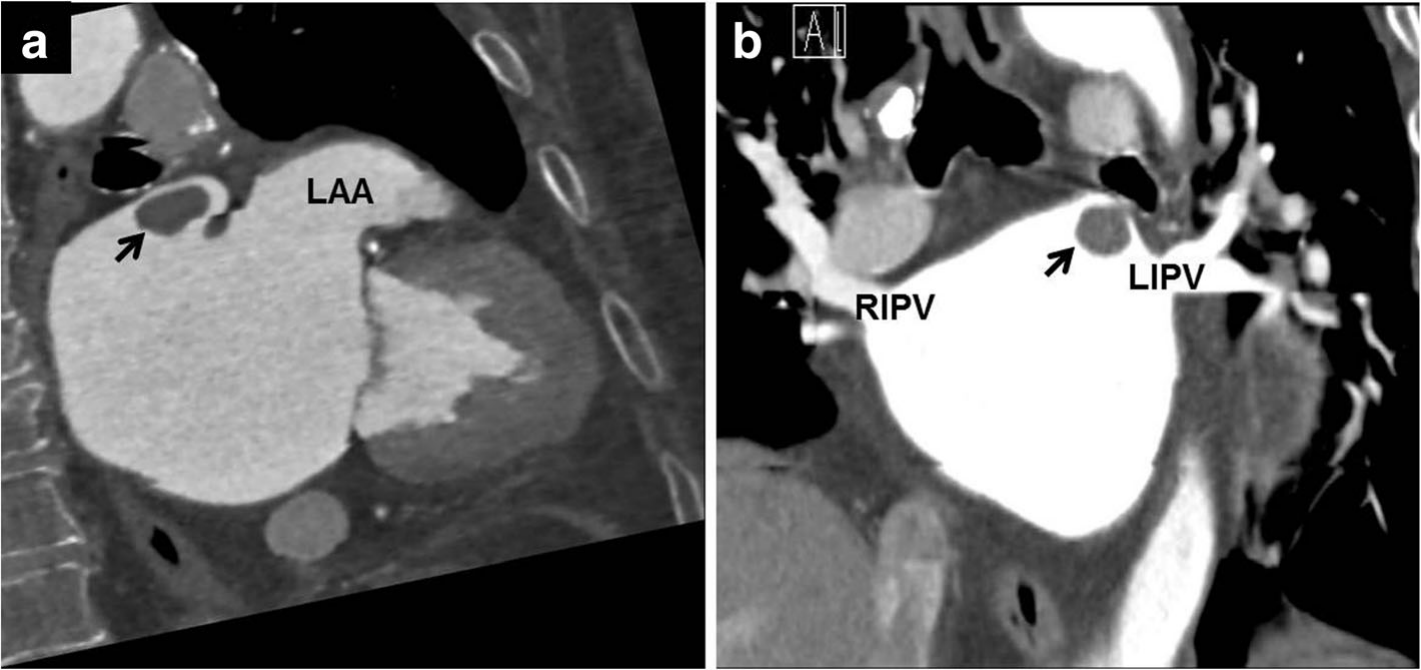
Cardiac computed tomographic angiography demonstrating about 1.6 × 1.4 cm sized thrombus within the remnant stump of the left superior pulmonary vein and no thrombi within left atrial appendage (LAA)(a, b). RIPV: right inferior pulmonary vein, LIPV: left inferior pulmonary vein
